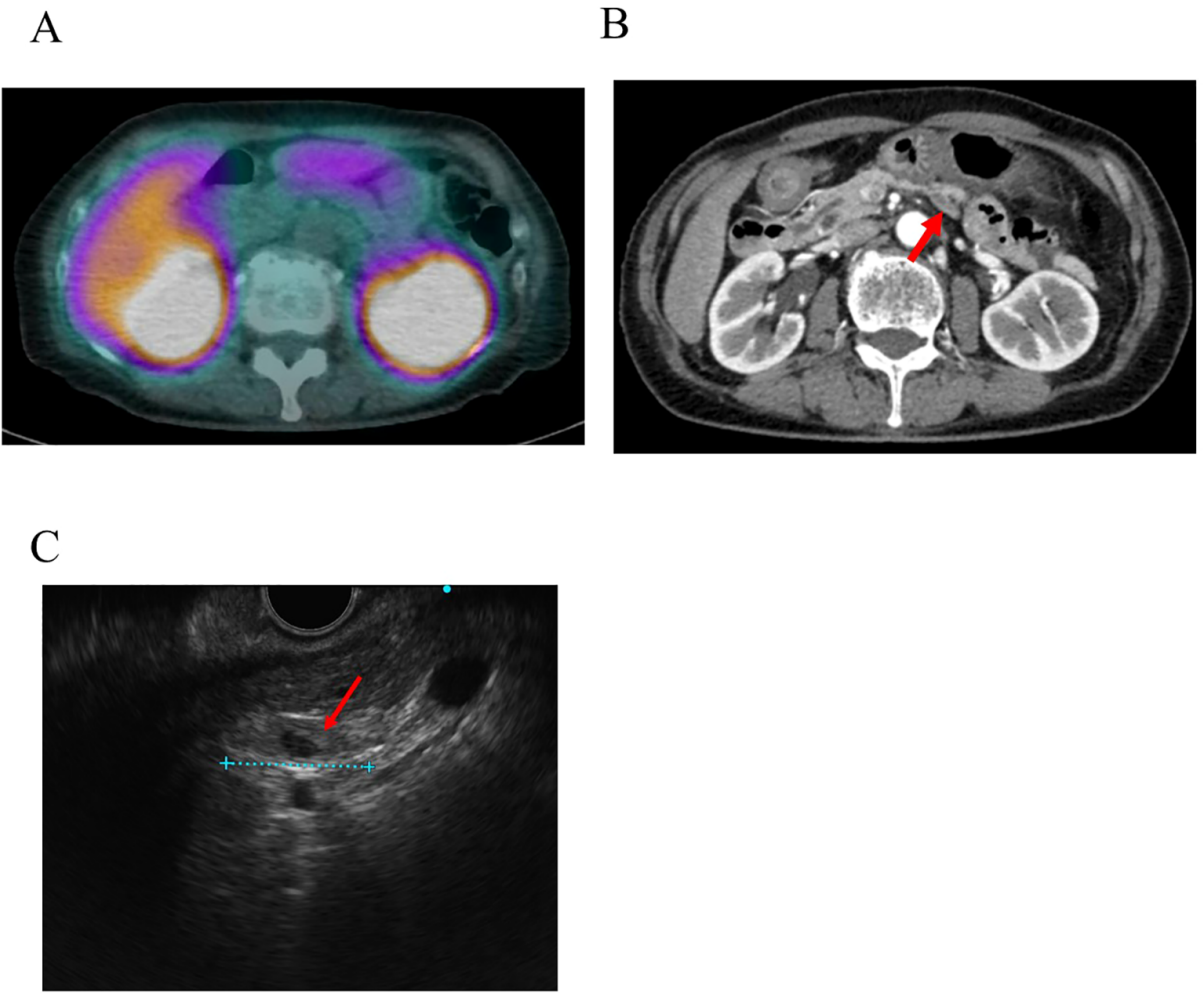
Images produced by conventional techniques. (A) [^111^In-DTPA-D-Phe]-octreotide single-photon emission CT (SPECT). No abnormal uptake was observed at the site where the tumor was later identified. (B) The nodule identified by [^18^F] FB (ePEG12)12-exendin-4 PET/CT results in contrast-enhanced CT. The red arrow indicates the nodule. (C) Nodule detected by endoscopic ultrasound (EUS) conducted after [^18^F] FB (ePEG12)12-exendin-4 PET/CT. The red arrow indicates the nodule.
